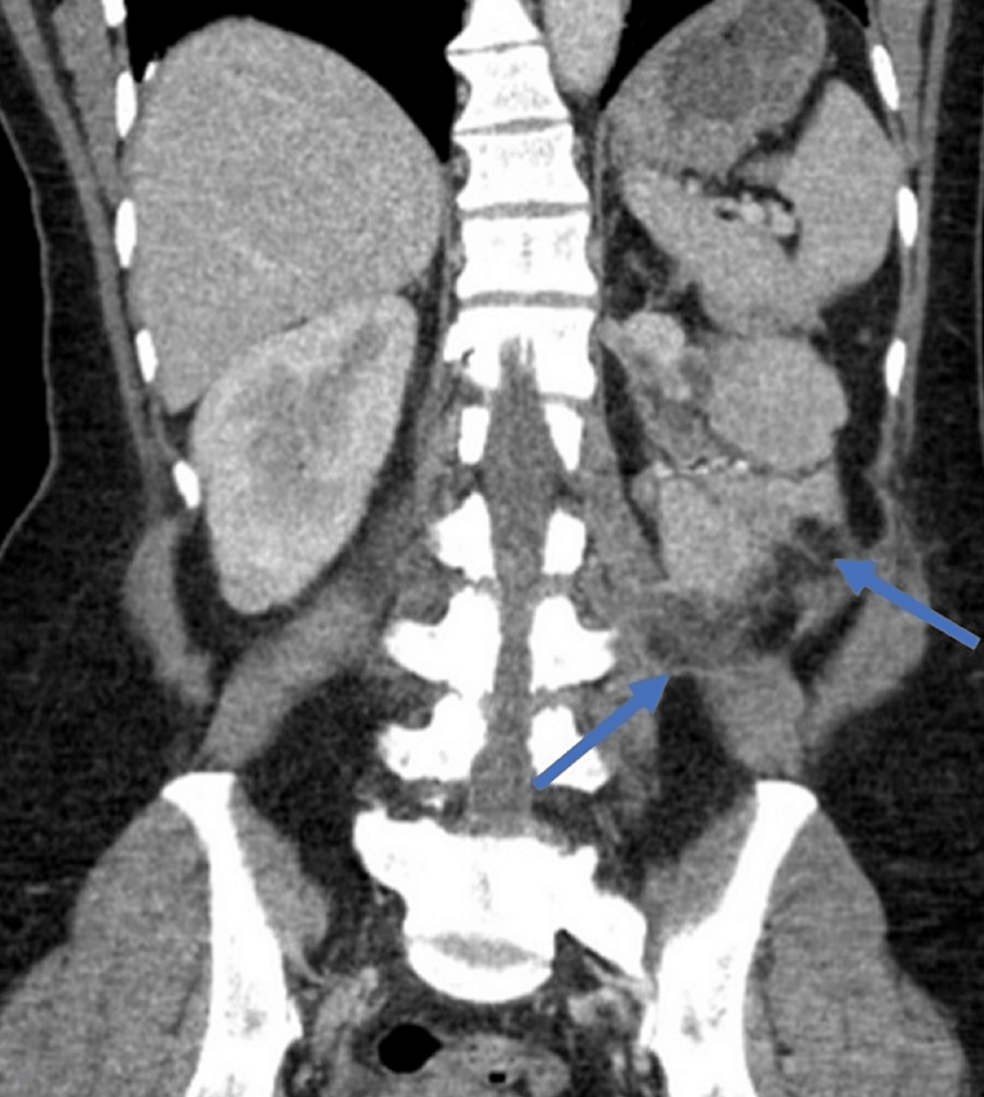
CT abdomen demonstrating an enhancing lesion in the left kidney (blue arrows).
